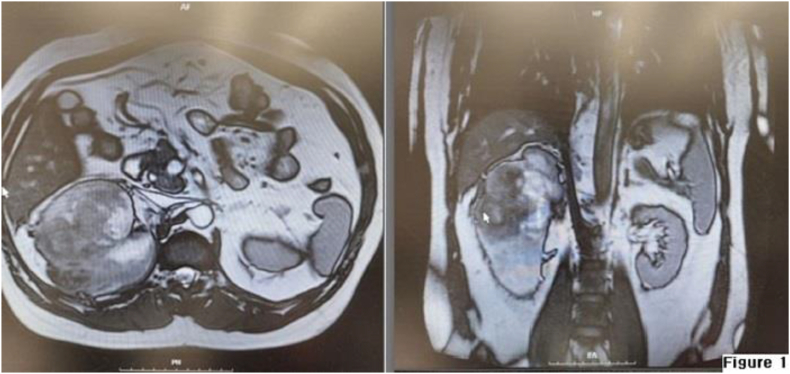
Abdominopelvic MRI showed 101 × 92 mm mass in upper-middle pole of right kidney.
